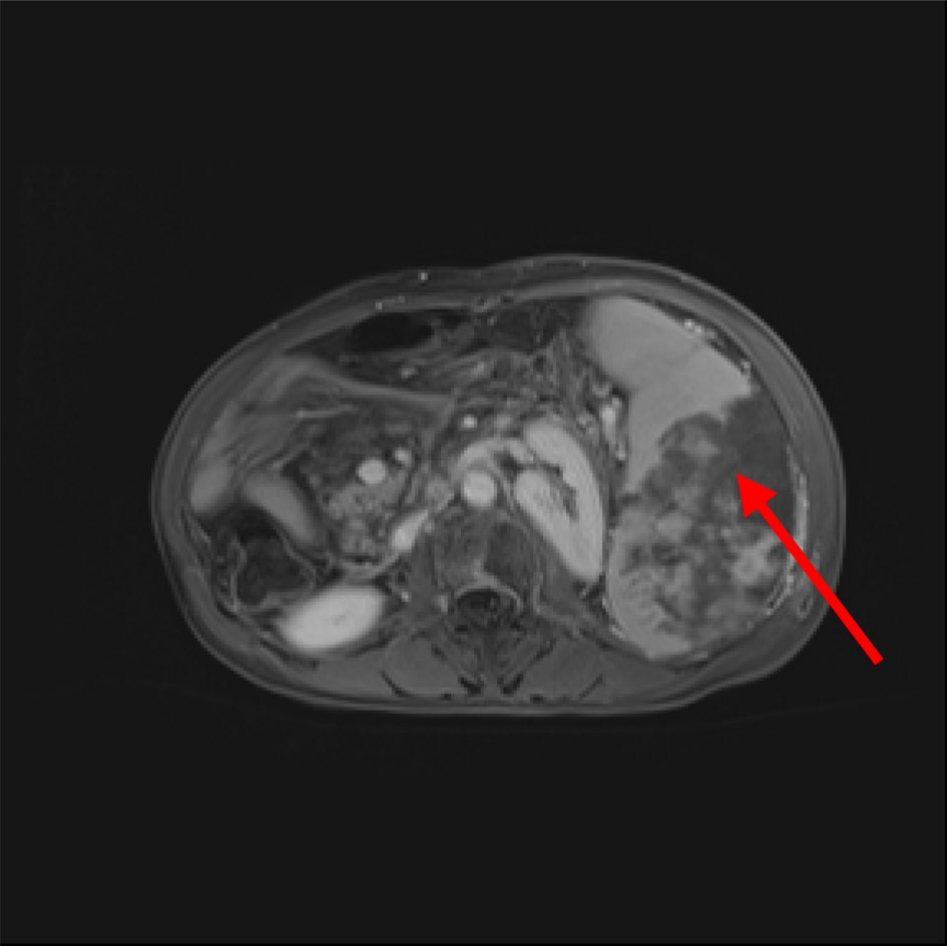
Contrasted MRI showed a patchy nonperfused area of the spleen two weeks after the HIFU treated region of the spleen (red arrow).
